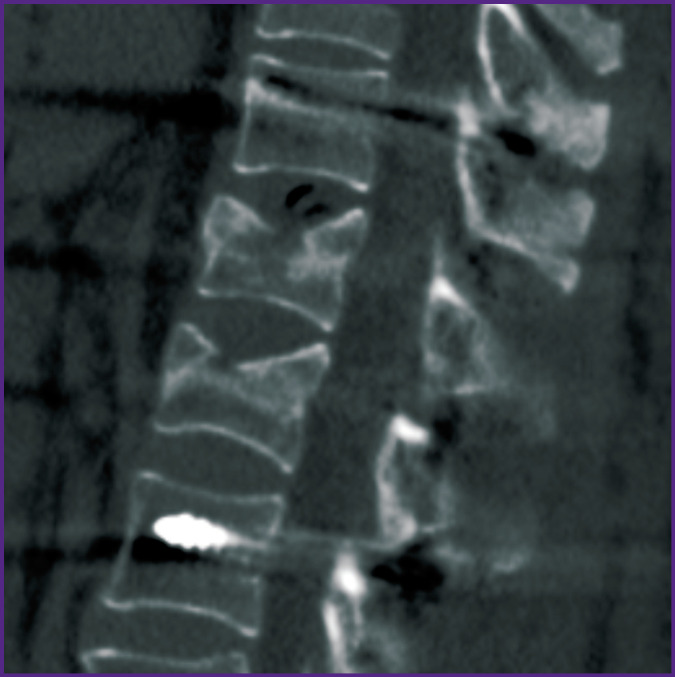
CT scan of Th_12_ and L_1_ vertebrae of patient I. 6 months after surgery
